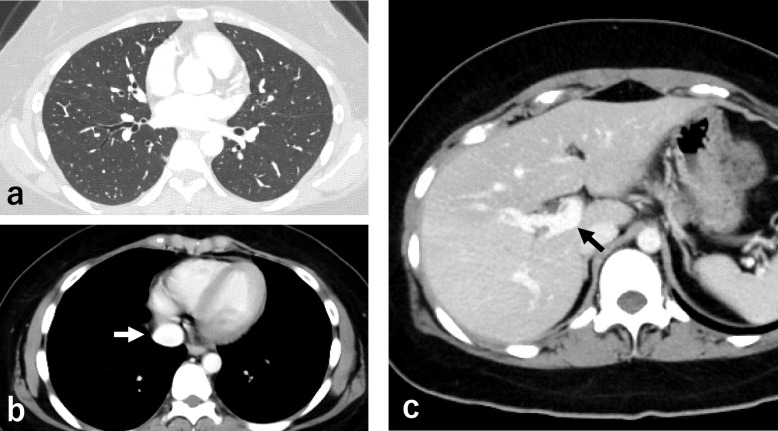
Chest and abdominal CT image findings after improvement. a CT scan on day 20 showing improvement in pulmonary infiltrates. b, c Contrast CT scan on day 40 showing a significant reduction in the size of multiple thrombi
